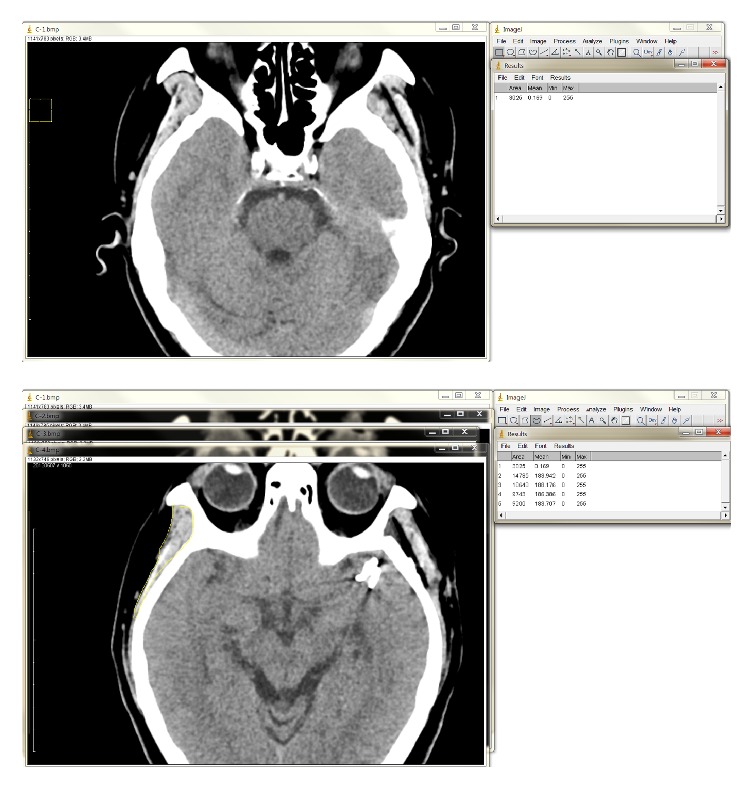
Calculation of temporalis muscle volume using ImageJ. (Top) A square with the length of the reduced scale (1cm) in an axial section of a computed tomography (CT) image was drawn. The number of pixels inside the square per unit area (1 cm^2^) was calculated to be 3025 using the rectangular selection tool. (Bottom) A curved line was drawn along the margin of the temporalis muscle using the freehand selection tool. The number of pixels inside the region of interest was determined.
